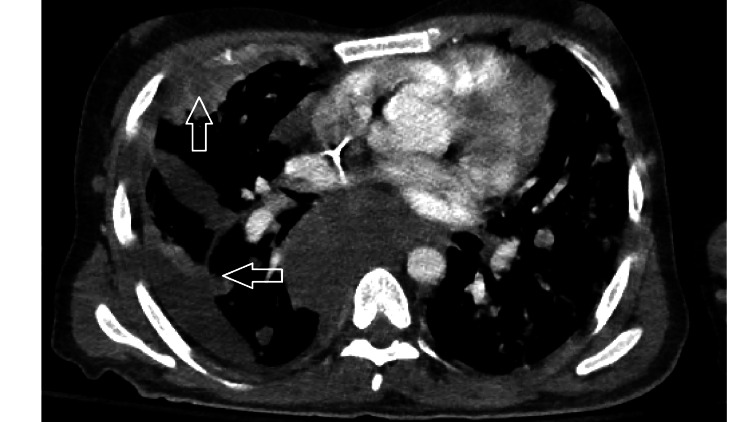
Pulmonary nodules and surrounding edema as seen on chest CT on day 2 of chemotherapy.
